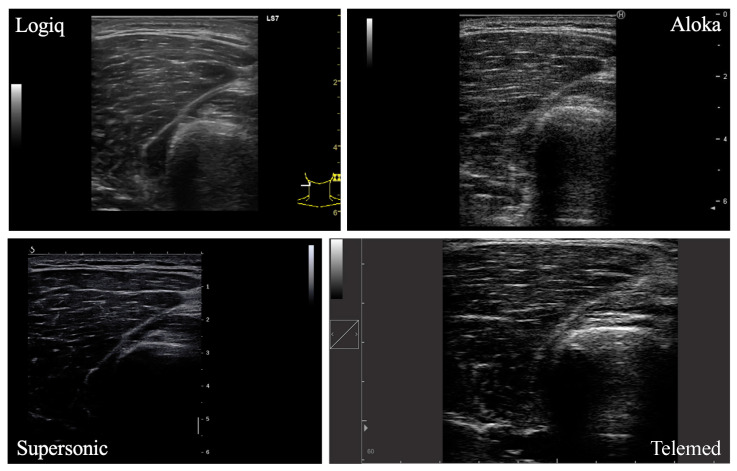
Ultrasound images of the vastus lateralis muscle from four different devices.
